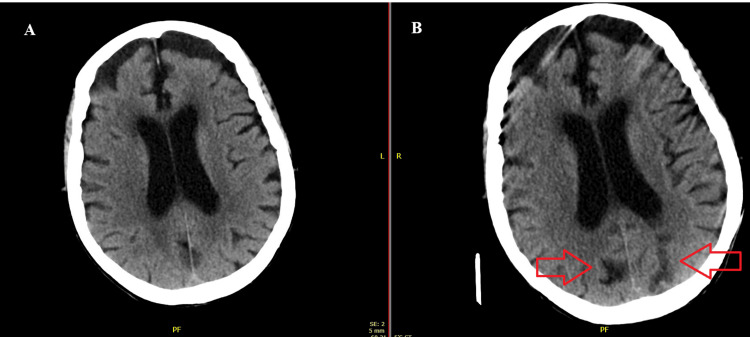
Initial CT head without contrast (A) vs subsequent CT head with and without contrast (B) with new subacute occipital infarct
